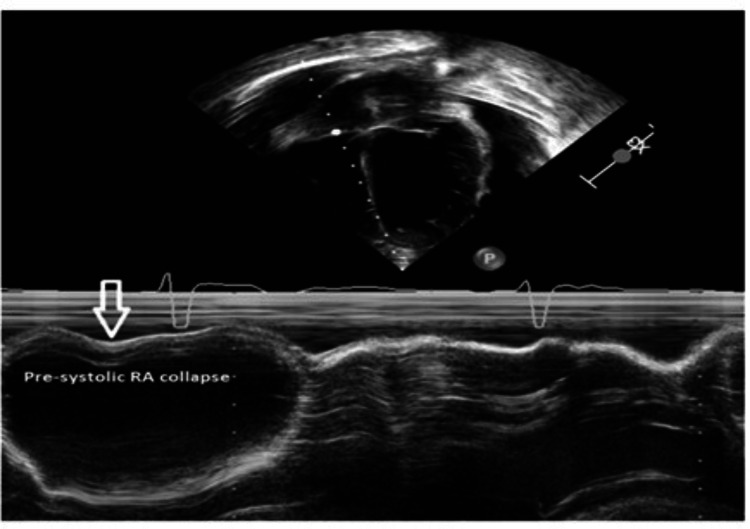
Initial echocardiography showing the pre-systolic right atrial collapse RA: right atrial.
